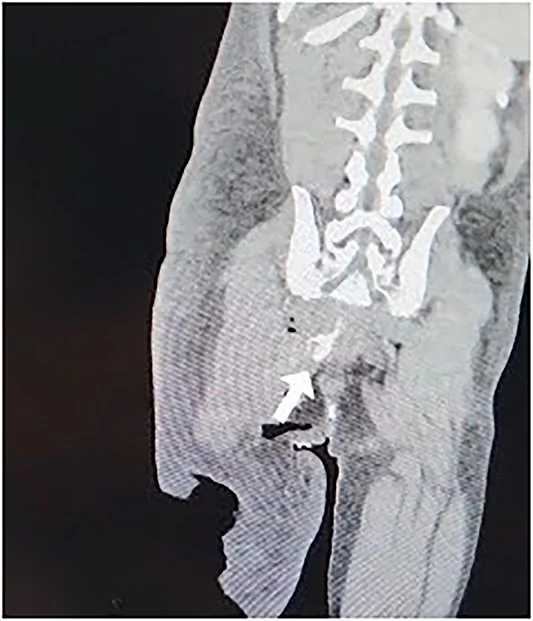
Coronal view of ischiorectal fistula as seen on CT abdomen and pelvis. Arrow: contrast blush identifying location of fistulous tract.
